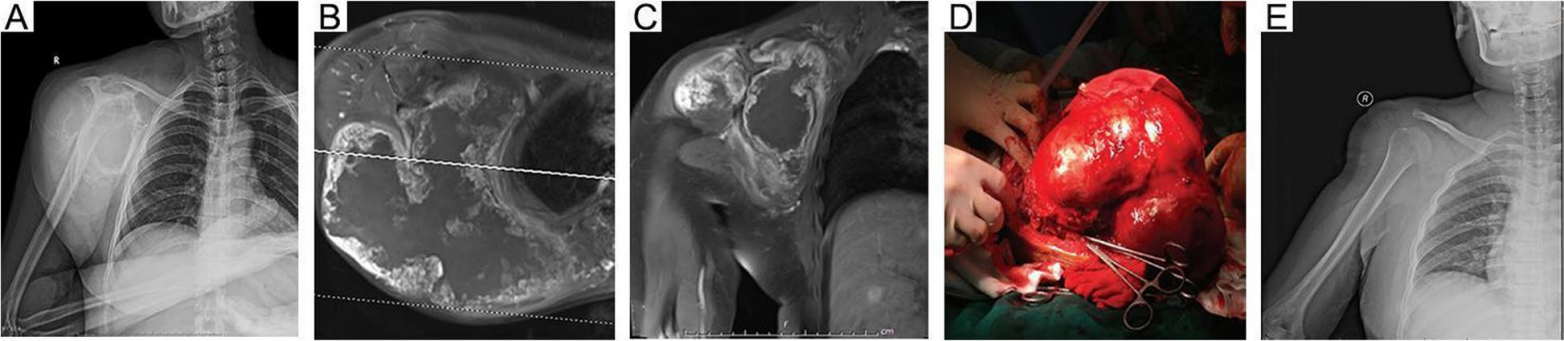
A 49-year-old female patient underwent total scapulectomy + humeral suspension for giant highly differentiated chondrosarcoma of the right scapula (case #4 in Table 1). A: X-ray imaging showing osteolytic destruction of the right scapula. B-C: MRI showed a massive tumor mass in the right shoulder (T1WI). D: Images of intraoperative tumors. E: Postoperative radiography showed that the shoulder was in good shape
